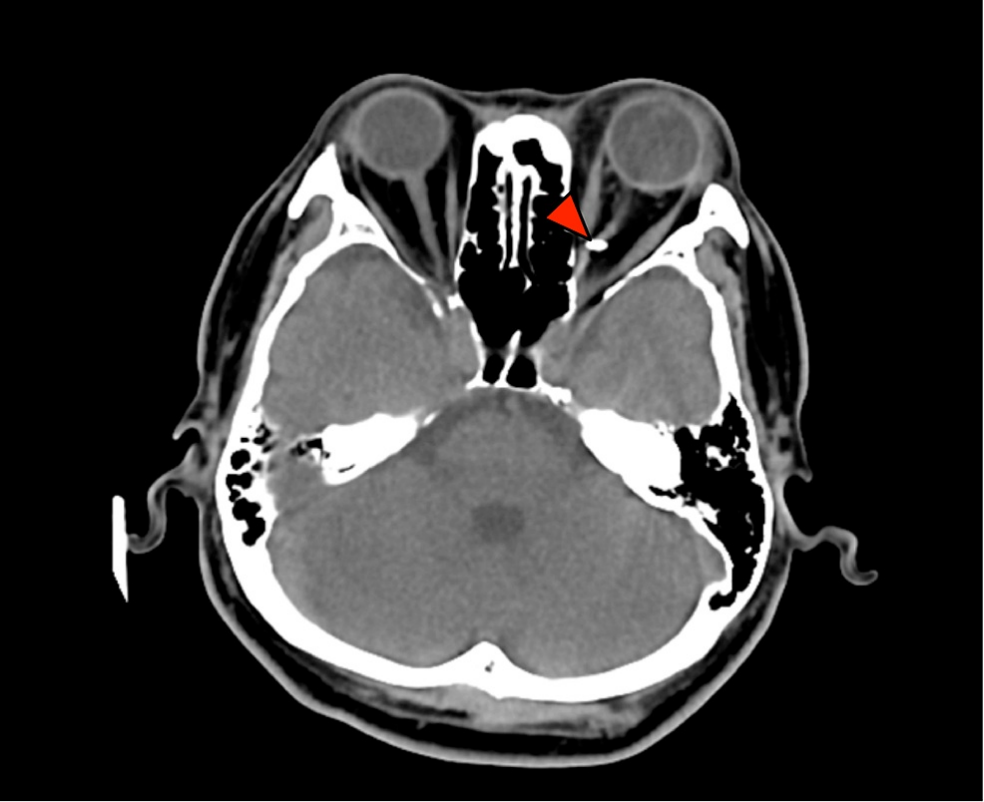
CT scan demonstrating a foreign body (arrow)
